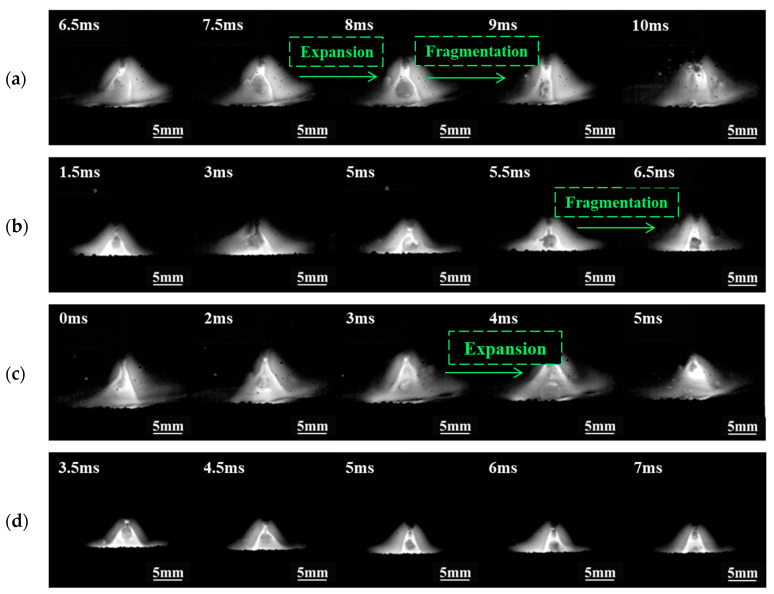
Influence of ultrasonic power on globular transfer. (a) 0.8 kW; (b) 1.2 kW; (c) 1.6 kW; (d) 2.0 kW.
